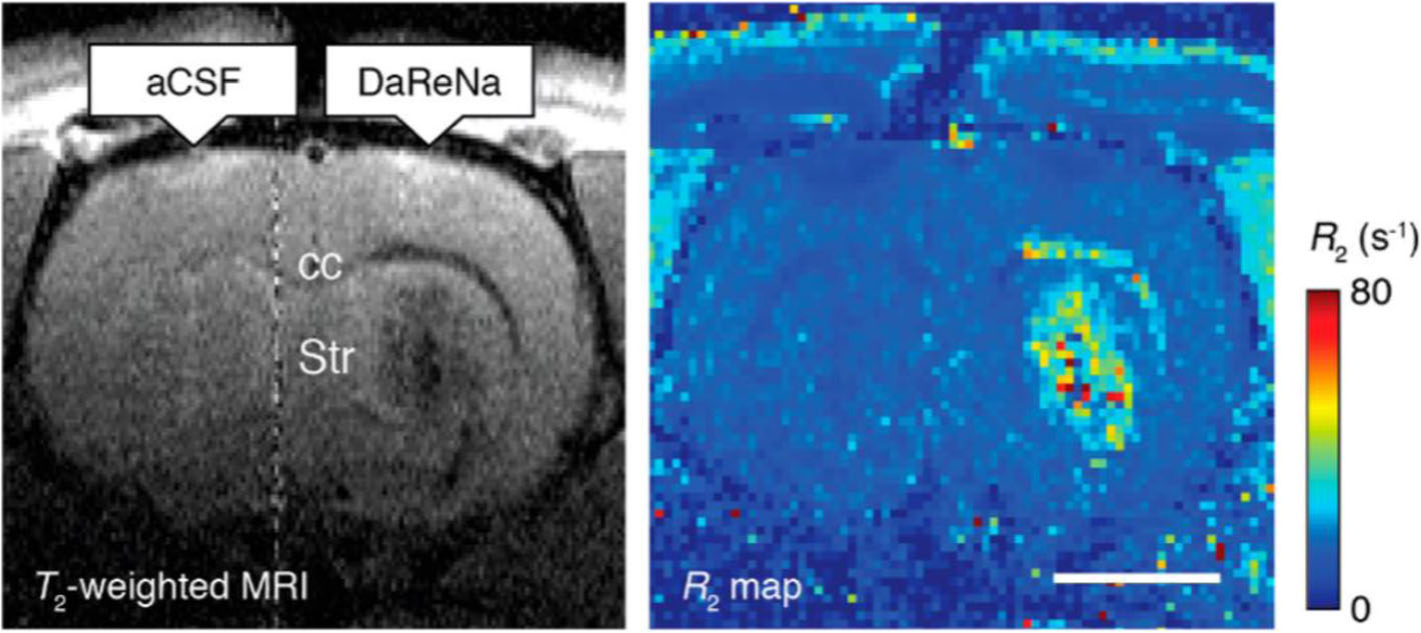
MRI contrast agent. Infusion of the DaReNa, a dopamine sensor (BM3h) coated SPION complex, show the enhancement of contrast and relaxation near the injection site (striatum, Str) and along the corpus callosum (cc). Scale bar = 2 mm. Reprinted with permission from *Hsieh et al.*
